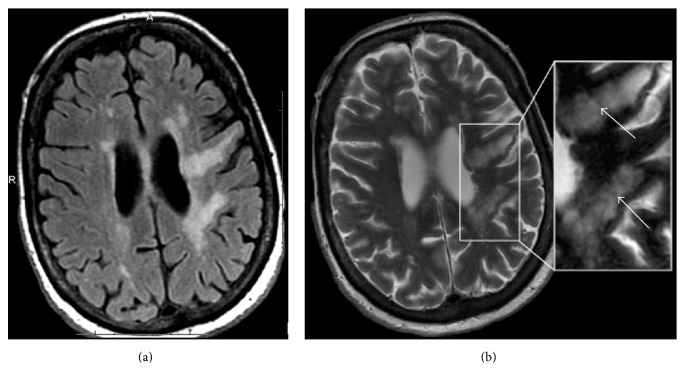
63-year-old woman with MS presenting with profound neurologic deterioration. (a) FLAIR image demonstrates characteristic PML lesions involving the subcortical U-fibers which also extent centrally to the periventricular surface. (b) T2-weighted image on the same patient demonstrates “granular” or “microcystic” foci (arrows).
